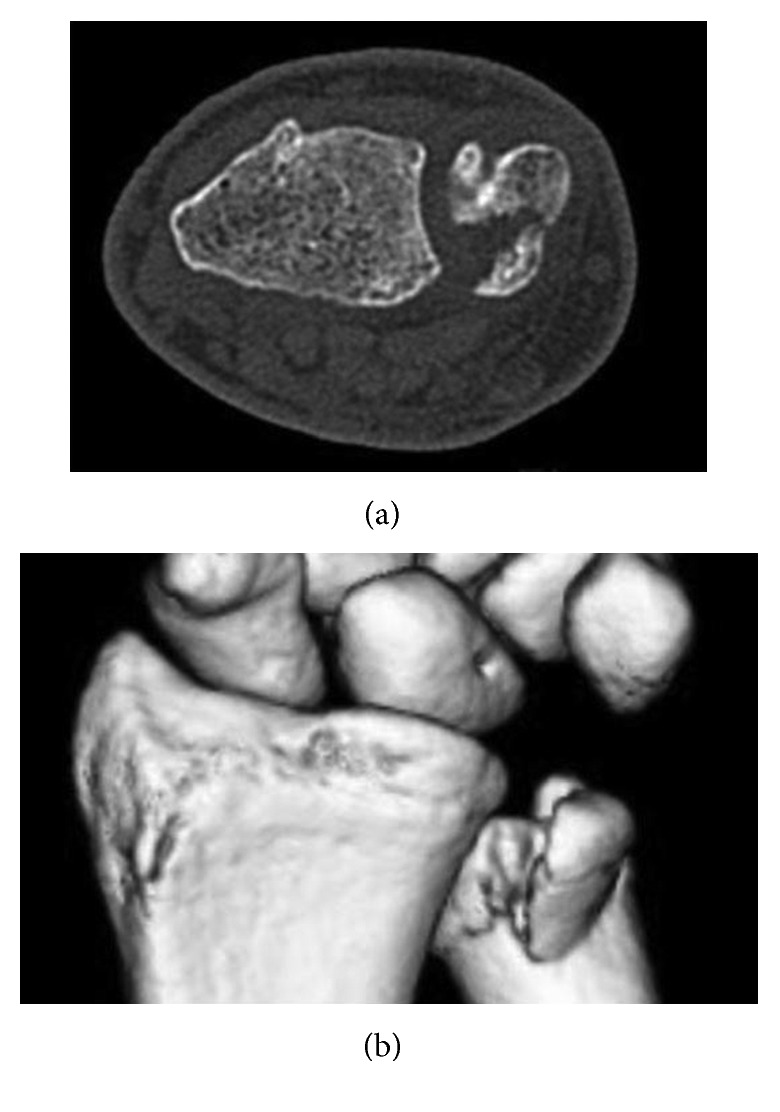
Preoperative CT in the supinated position. (a) Axial view and (b) 3D reconstruction.
